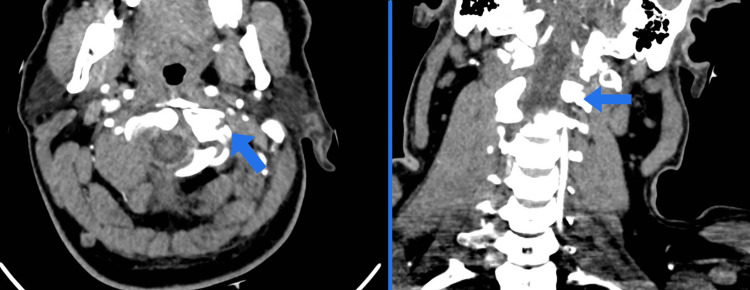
CT neck angiography for the upper cervical spine: the left vertebral artery is close to the fracture with no evidence of extravasation. CT, computed tomography
